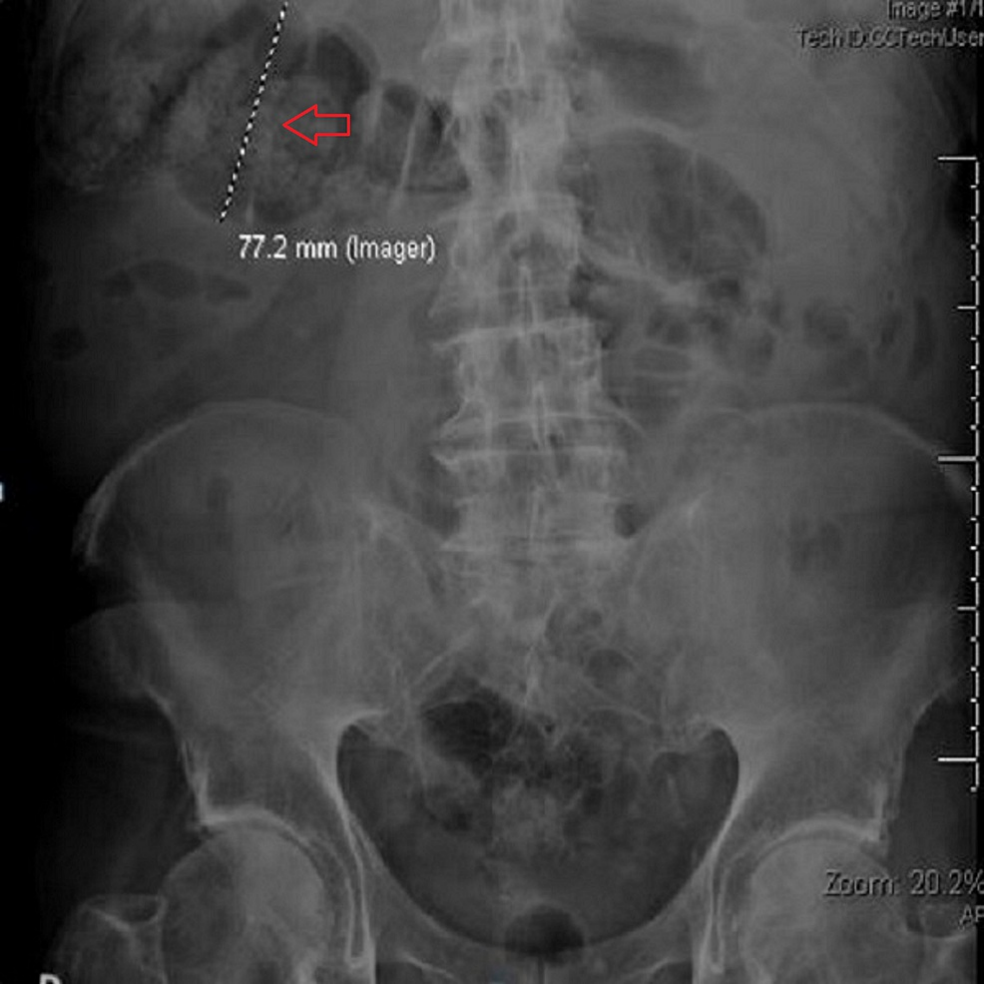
Abdominal X-ray of our patient. Red arrow: Dilated transverse colon, 7.7 cm in diameter.
